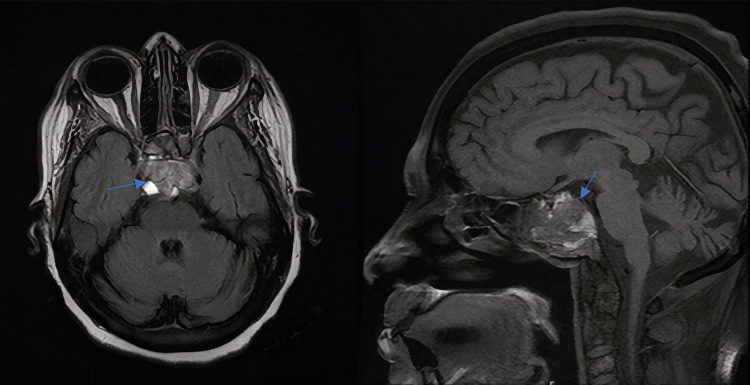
Axial and sagittal views of multiplanar and multisequential brain/orbits MRI with IV gadolinium contrast showing large expansile heterogeneously enhancing complex solid and cystic destructive mass lesion (arrows) involving the central skull base with the destruction of the clivus and extension into the bilateral sphenoid sinuses with no intracranial parenchymal lesions.
